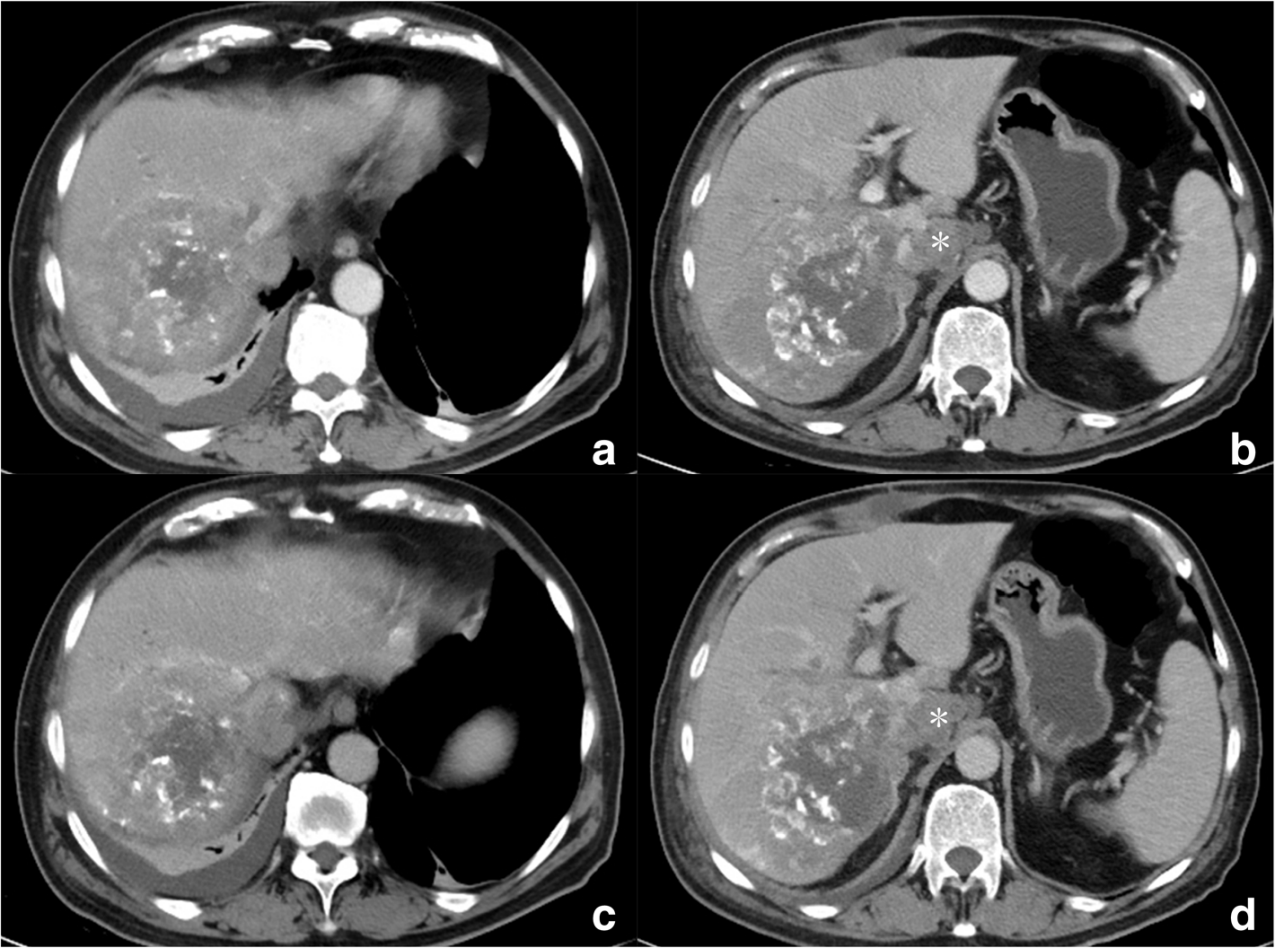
Contrast enhanced CT images of patient 6 who underwent TACE before surgery. a, b reveals a heterogeneous hypointense mass in right lobe with unclear rim, hyperdense iodine deposition secondary to TACE, and mild enhancement are identified in peripheral portion; c, d The enhanced portion of the tumor in AP demonstrated washout in PP, the border between tumor and the right adrenal gland is unclear, indicating involvement of right adrenal gland. Hepatic hilar lymphadenopathy is also presented (b, d.*)
